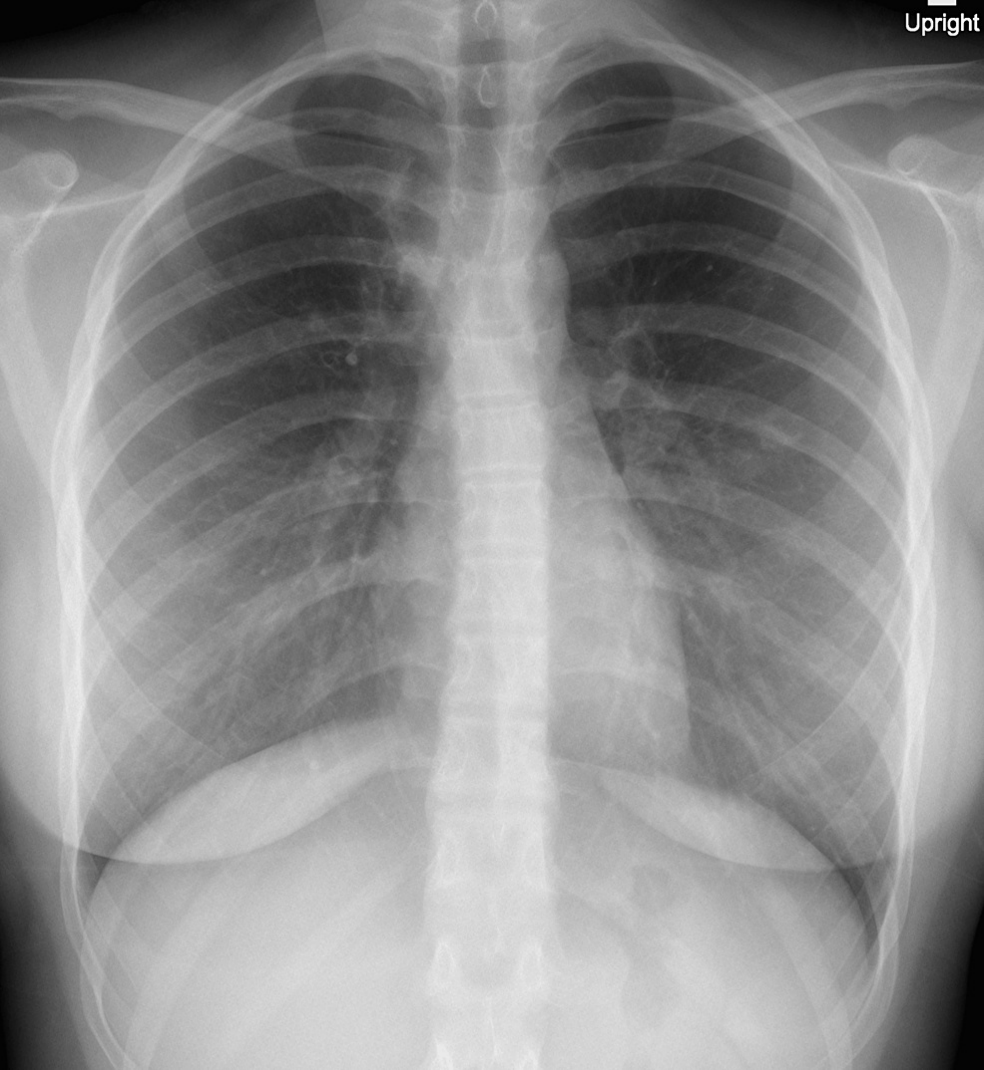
Chest X-ray showed improvement, with a significant reduction of the hilar mediastinal mass and resolution of parenchymal nodular infiltrates.
